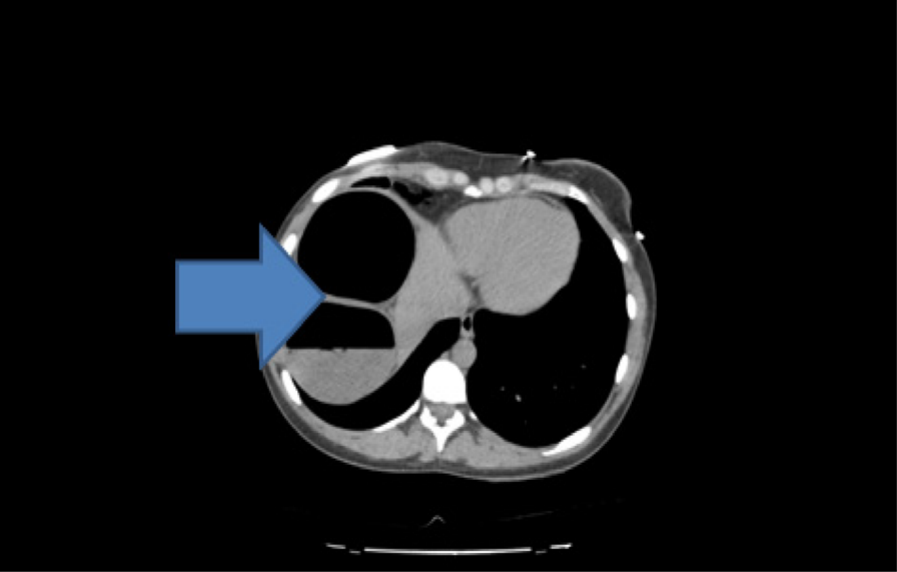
Computed tomography scan of the abdomen showing massive dilatation of the caecum, which measured greater than 14 cm in diameter.
